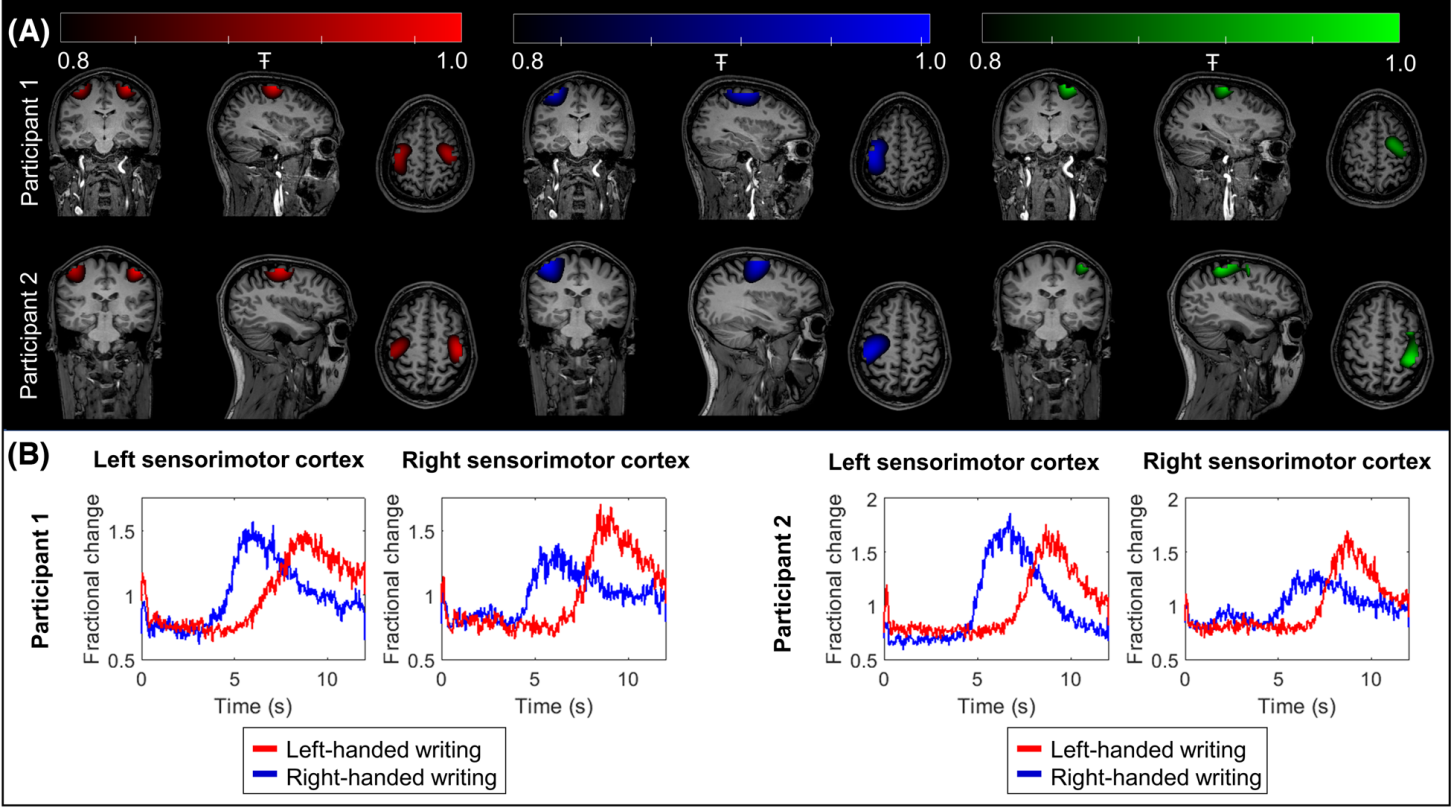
Brain function during left‐ and right‐handed writing. (A) Pseudo‐T‐statistical images, averaged across runs, thresholded at 80% of the maximum value, and overlaid on anatomical MRI. Activity during left‐handed trials is shown in red (left panel), right‐handed trials shown in blue (center panel), and the difference shown in green (right panel). Images for participant 1 are shown on the top row and participant 2 on the bottom row. For right‐ (dominant) handed writing, activity is dominant in the left motor region, whereas for left‐handed writing, a bilateral response is apparent. (B) The amplitude envelope of beta‐band activity in left and right sensorimotor cortices. Data from participant 1 are shown to the left, and participant 2 are shown on the right. For each participant, the graph to the left shows the left sensory cortex, and the plot to the right shows the right sensory cortex. In all cases, the blue traces show average (across runs) beta envelope modulation for right‐handed writing, and red shows the same for left‐handed writing.
